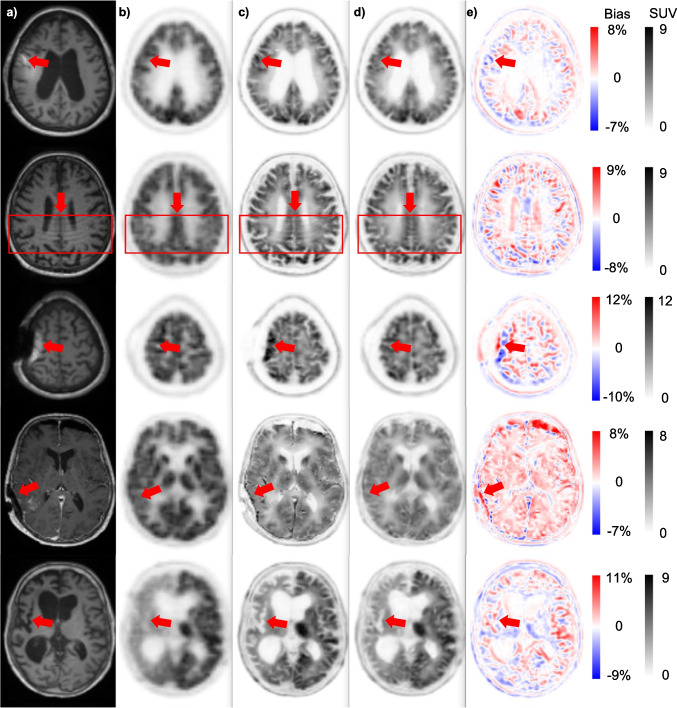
Five special cases of patients presenting with artifacts in MR images (first and second row), anatomical abnormalities or existence of external objects (third and fourth row), and ununiform activity distribution in PET images (last row): (a) coregistered T1-weighted MRI, (b) non-PVC PET images, (c) reference corresponding MRI-guided PVC PET images, (d) DL-predicted PVC PET images, and (e) the corresponding bias maps
